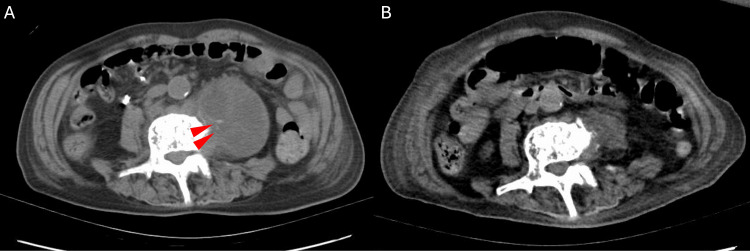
Abdominal computed tomography on admission and five months after the admission. (A) Abdominal computed tomography on admission revealing abscess with calcification (arrowheads) in the left psoas muscle. (B) Abdominal computed tomography five months after the admission showing abscess improvement.
